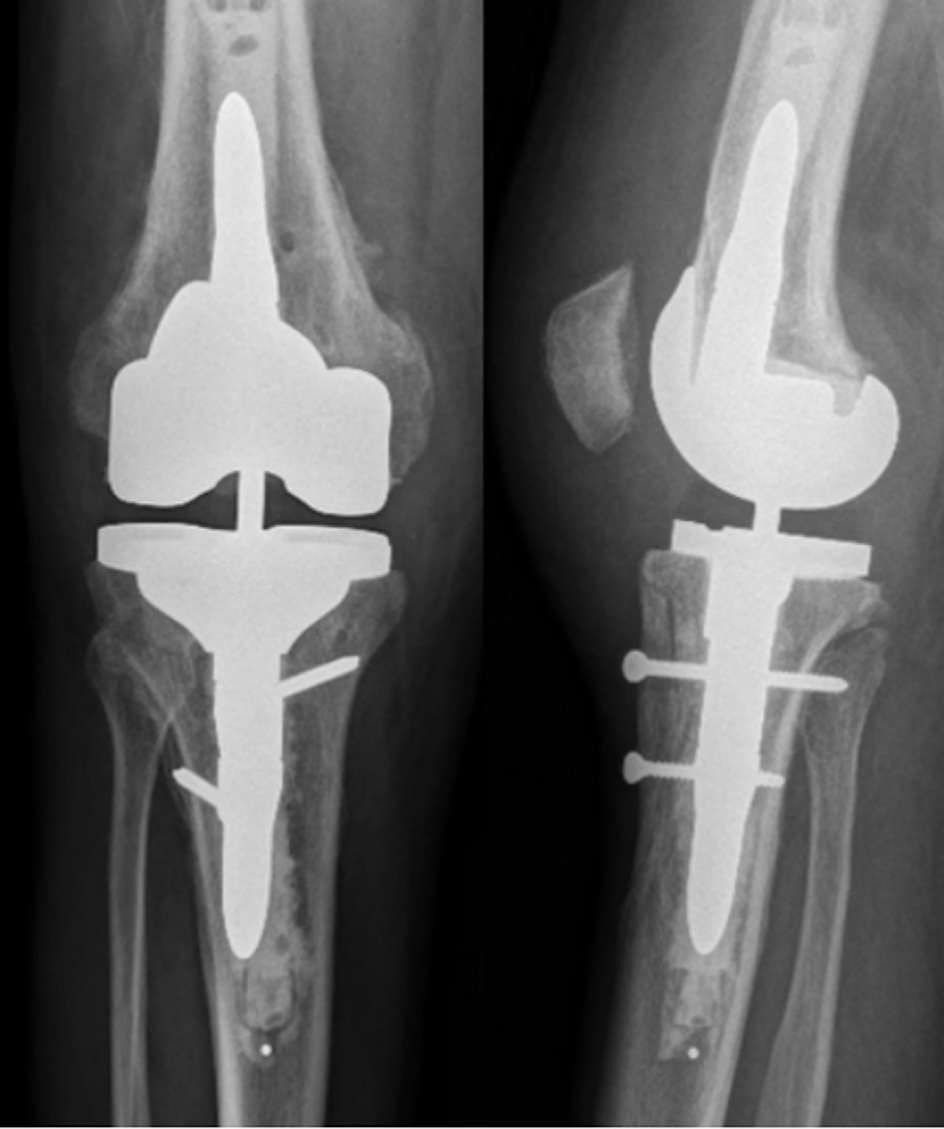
X-ray control at 3 months, confirmed bone union.
